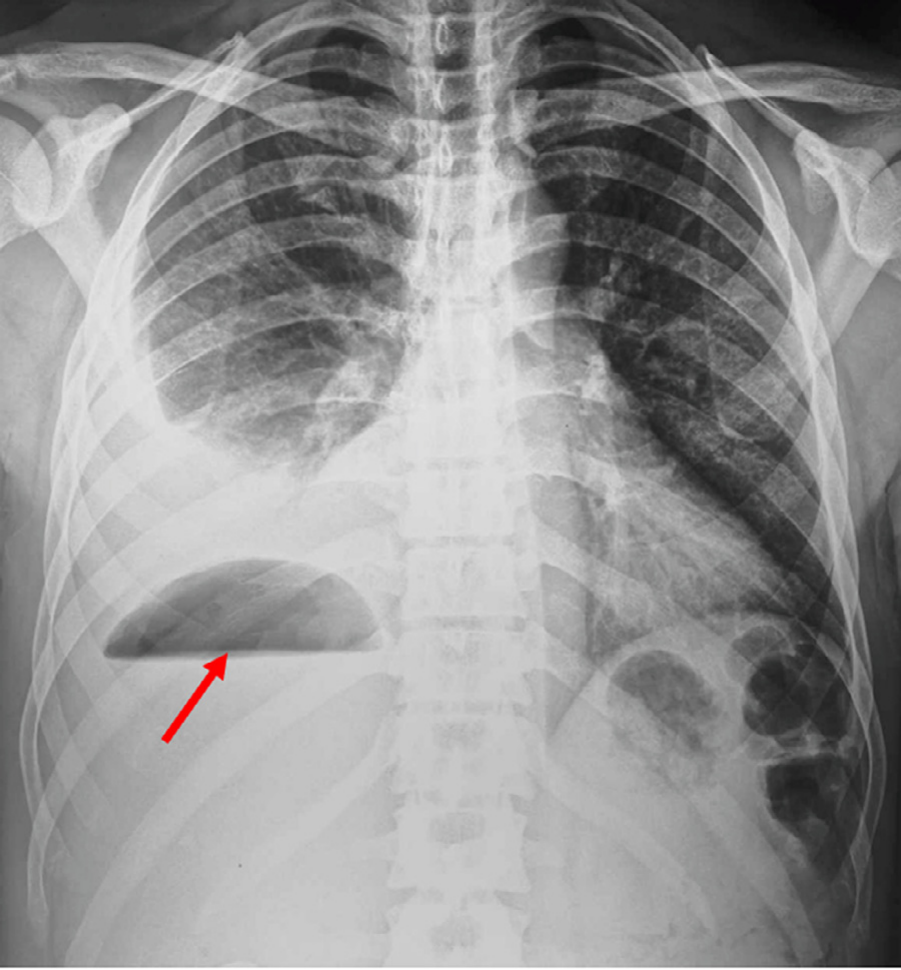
Chest and abdominal X-ray showing the hydro-aeric level in the upper right quadrum of the abdomen (red arrow).
